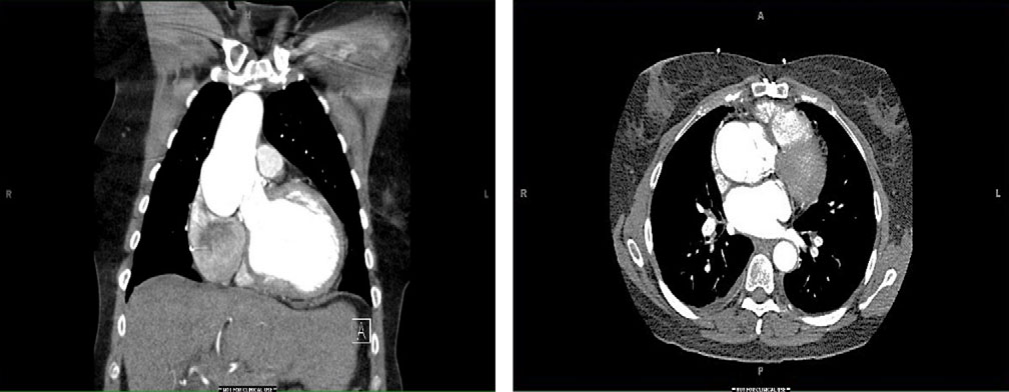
CT of the chest. Coronal (left) and axial (right) views demonstrating pathologic dilatation of the aortic root and ascending aorta. On the axial image, a dissection plane is noted in the aortic root.
